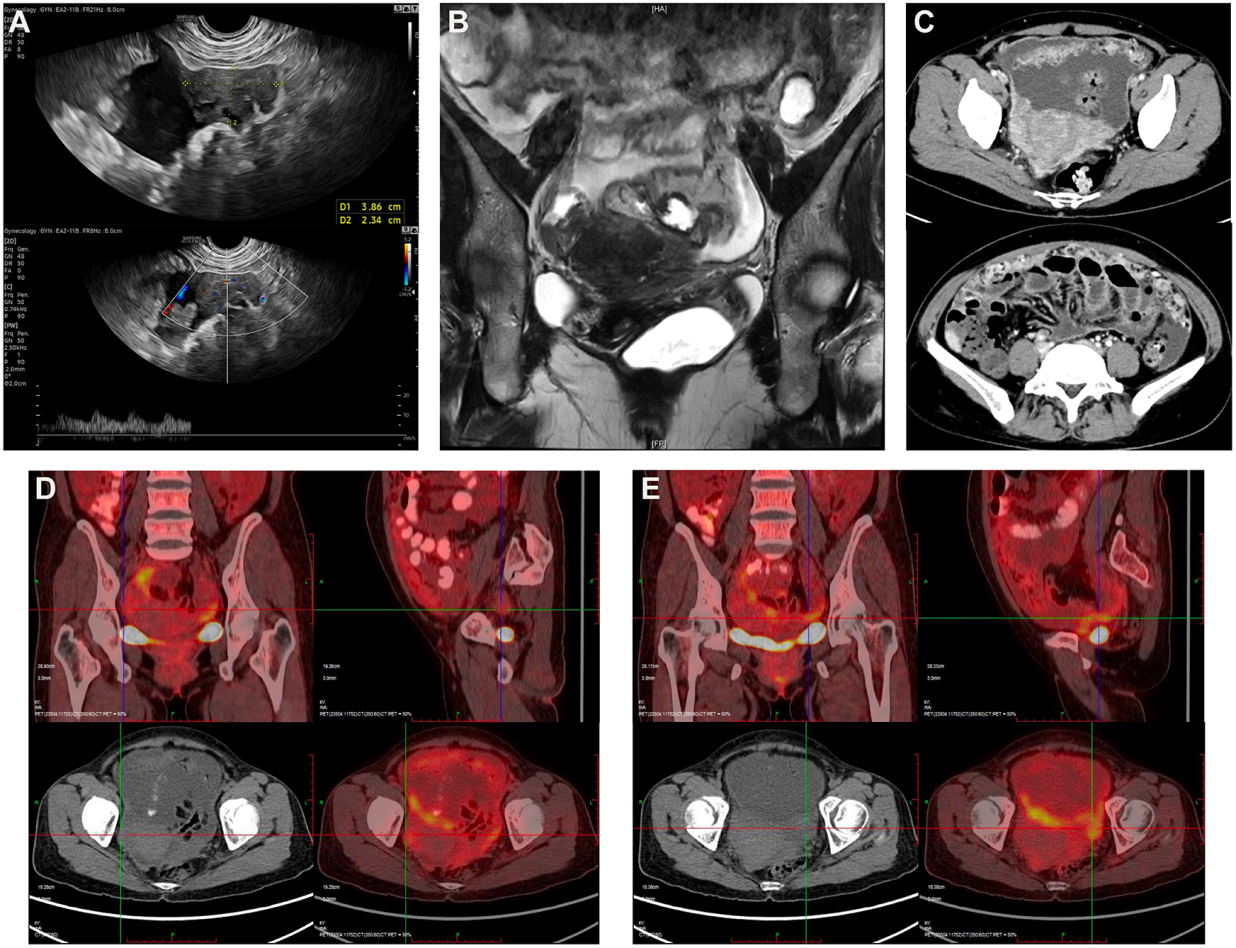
(A) Transvaginal ultrasound reveals a 3.9×2.3cm irregular hypoechoic mass in the right abdominal cavity with blood flow signals closely related to the peritoneum. (B) Pelvic MRI shows a suspicious mass in the right adnexal area and thickening of the omentum with pelvic effusion. (C) Abdominal and pelvic enhanced CT reveals plump bilateral adnexal masses, diffusely thickened peritoneum, omentum, and mesangium, slightly thickened intestinal wall, and abdominal and pelvic effusion. PET-CT displays plump bilateral adnexal areas, poorly demarcated from surrounding tissue, showing signs of hypermetabolism with (D) the SUVmax2.2 on the right and (E) the SUVmax3.1 on the left.
